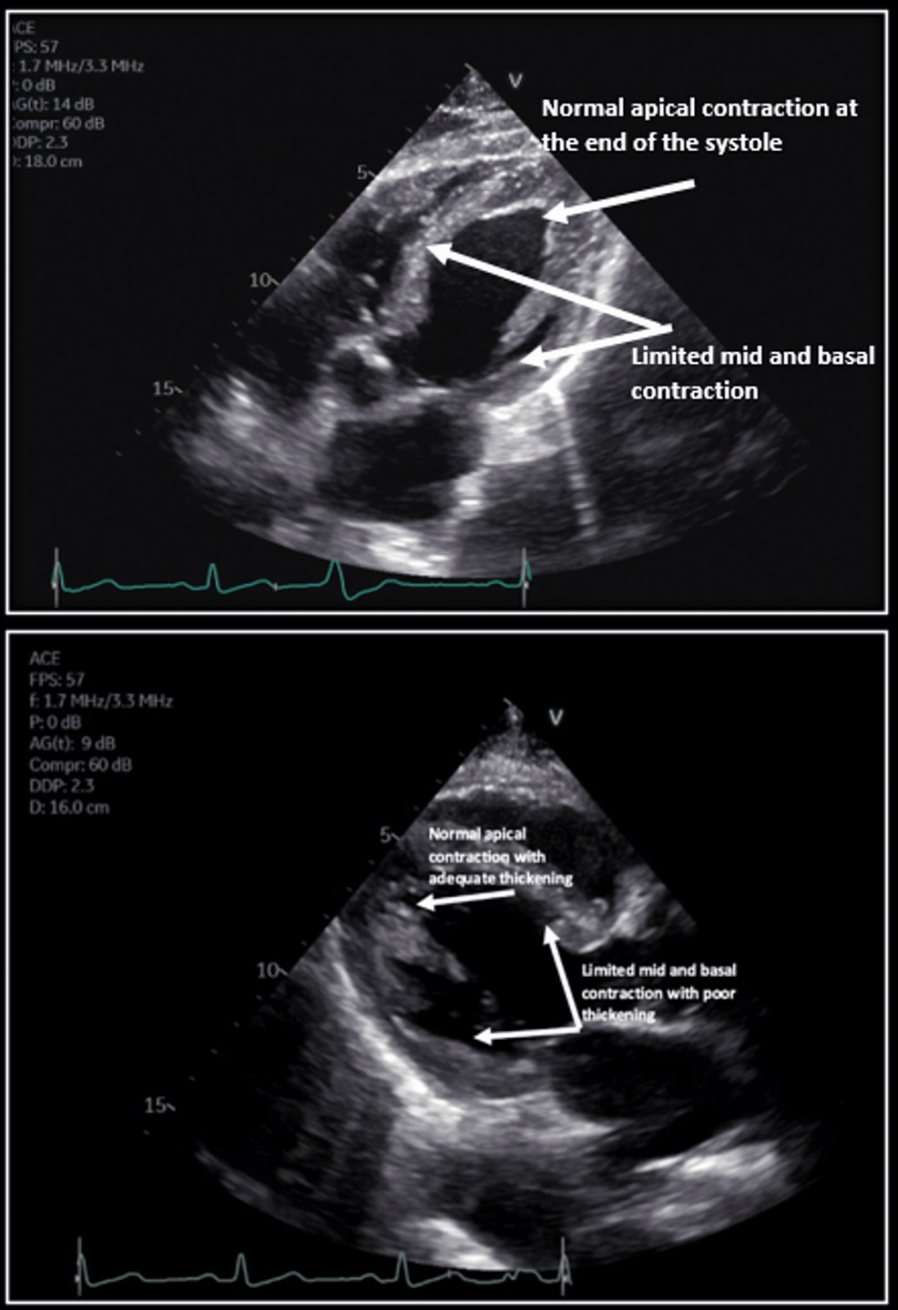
Transthoracic echocardiogram, apical-four chamber view (upper photo), parasternal long axis view (lower photo) at the end of the systole showing fully contracting apex and limited basal and mid segments contraction.
